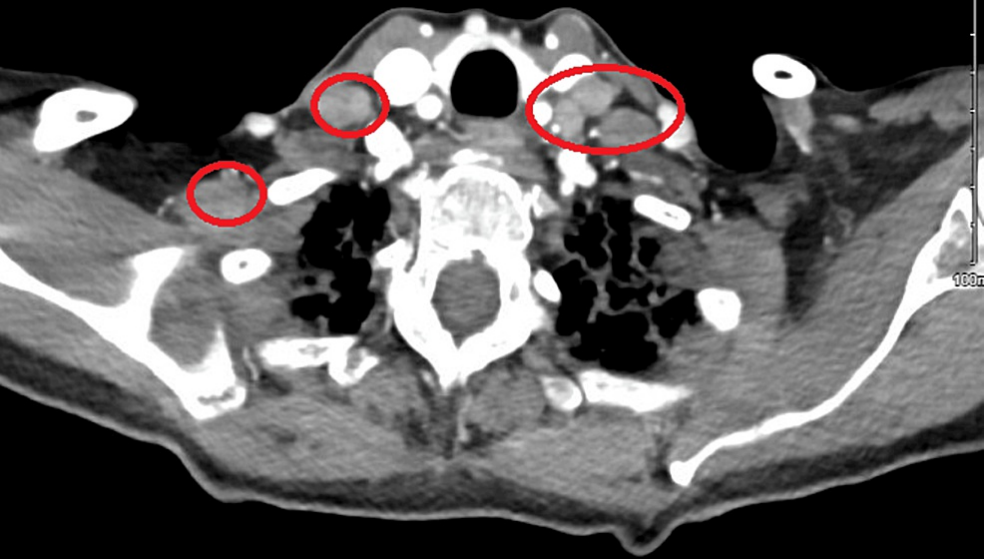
CT neck showed multiple pathologically enlarged and/or pathologic appearing lymph nodes. Supraclavicular lymph node also identified.
